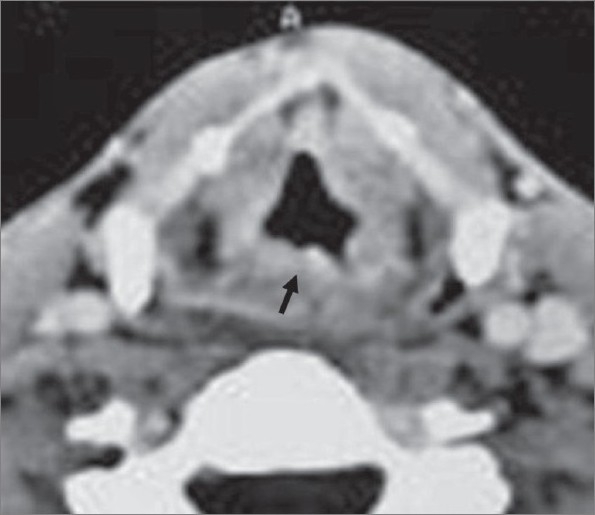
Axial contrast-enhanced CT scan of the larynx shows irregular thickening of the posterior laryngeal wall (arrow)
